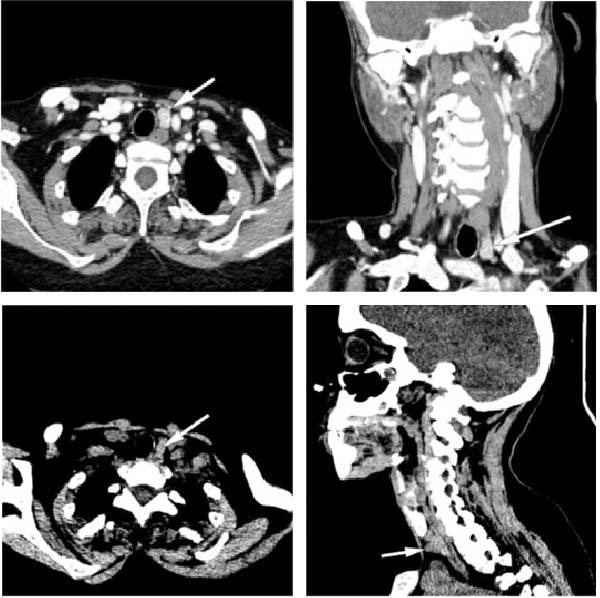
Contrast‐enhanced CT of the neck (axial, coronal and sagittal reconstructions) demonstrates a well‐defined, avidly enhancing lesion measuring approximately 1.3 cm × 2.0 cm, located posterior to the left thyroid lobe, consistent with a left‐sided parathyroid adenoma.
